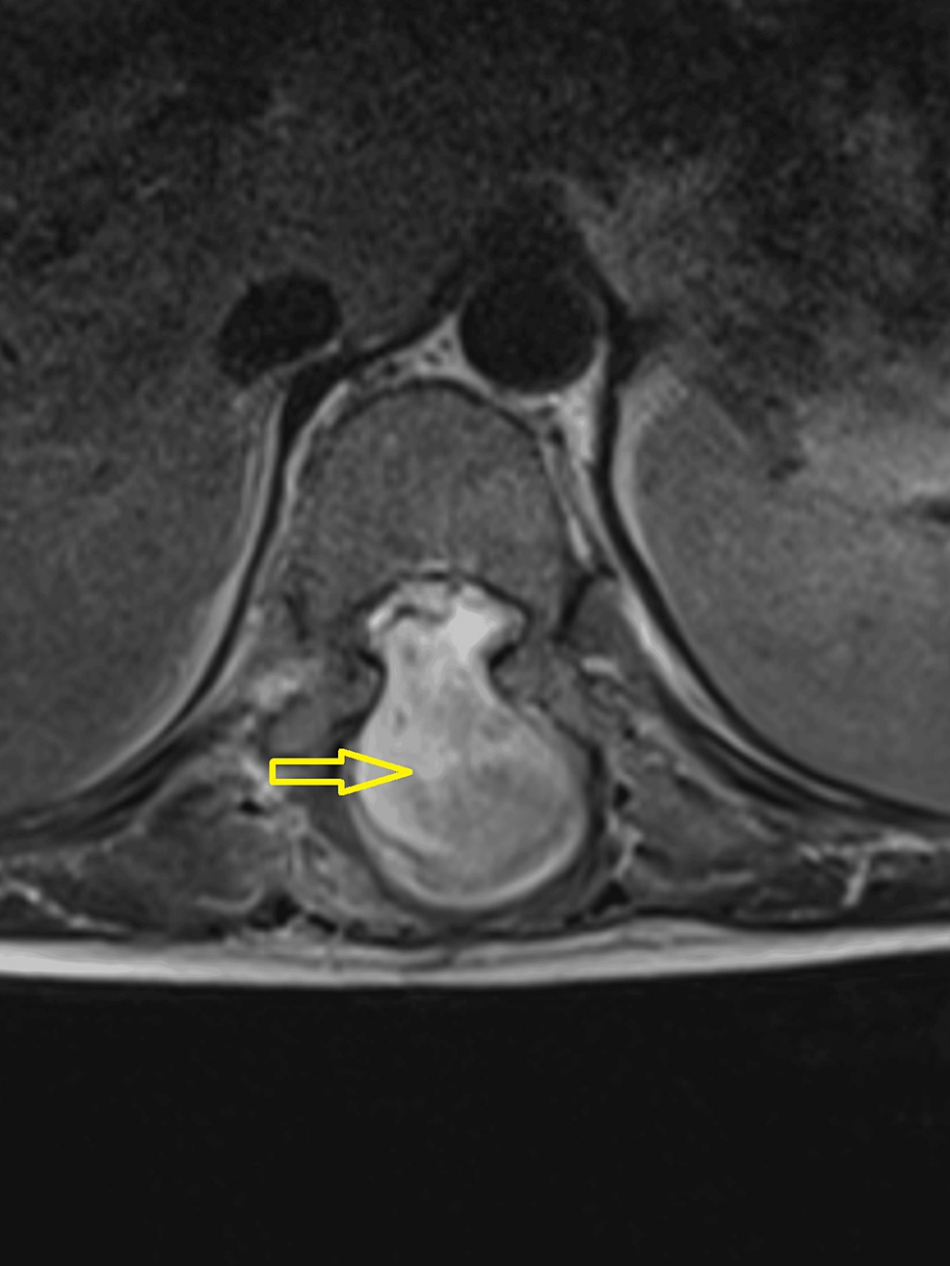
An MRI of the thoracolumbar spine (axial images) Features of arachnoiditis, loculated cerebrospinal fluid (CSF) collection, and dorsal spinal cord herniation with effacement of all the neural tissue (yellow arrow) are seen.
